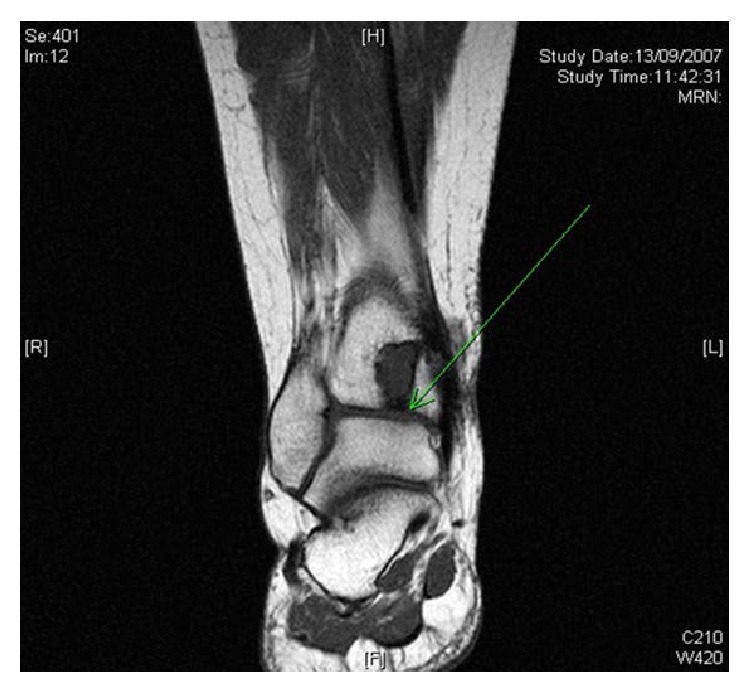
T1 weighted MRI showing 1.5 cm intracortical, subarticular cyst.
